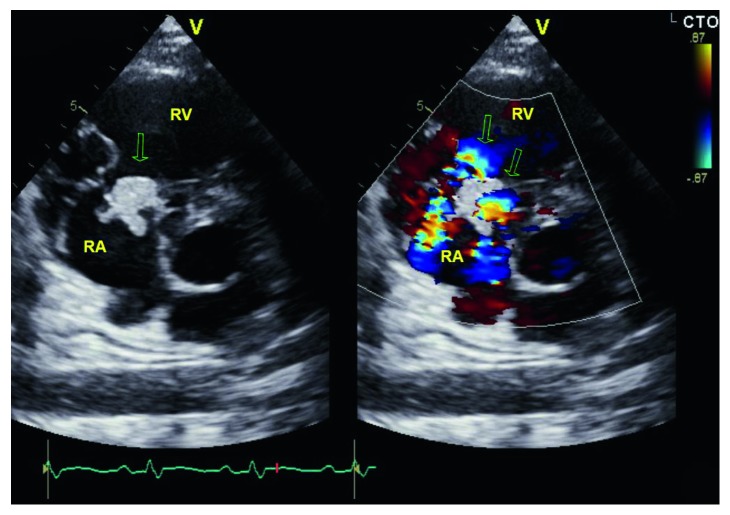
Parasternal short access view color flow Doppler showing the tricuspid regurgitation jet off axis and vegetation.
